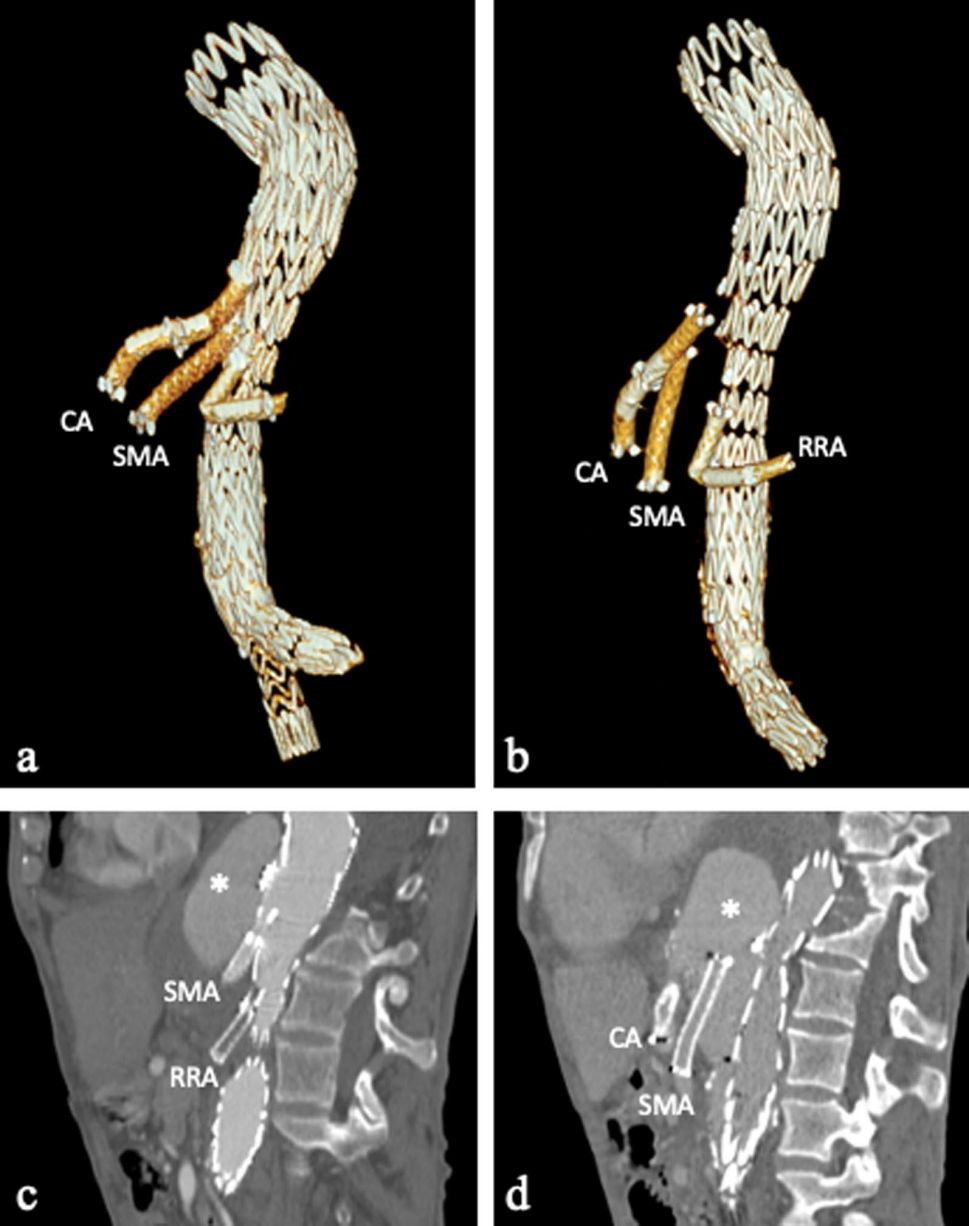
78-year-old man with stents migration. a Volume rendering reconstruction and b sagittal oblique CT images show a thoracoabdominal aneurysm treated with a f/b EVAR and three stent-grafts placement in aortic branches: celiac artery (CA), superior mesenteric artery (SMA) and right renal artery (RRA); the last one appears occluded. c Volume rendering and (d) sagittal oblique CT images after 3 years show distal migration of all three stent-grafts. In both examinations, there is contrast agent inside the aneurismal sac (*), likely a *type IIIb* endoleak due to imperfect junction of the branches with the main device body
